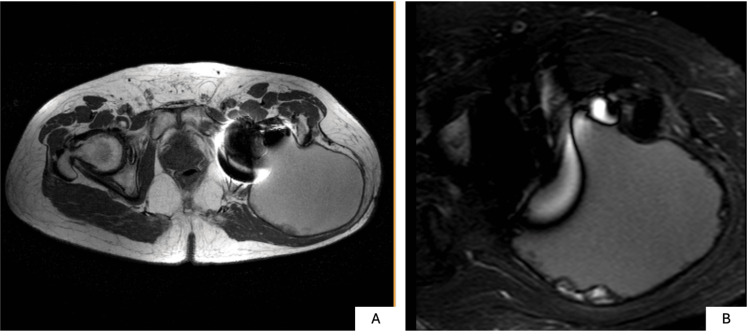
Axial MRI of the left hip obtained using metal artifact reduction sequences (MARS) (A) T1-weighted MARS image shows a well-circumscribed lesion with predominantly low to intermediate signal intensity. (B) Short tau inversion recovery (STIR) MARS image demonstrates marked hyperintensity consistent with a fluid-containing lesion, supporting the diagnosis of chronic expanding hematoma.
